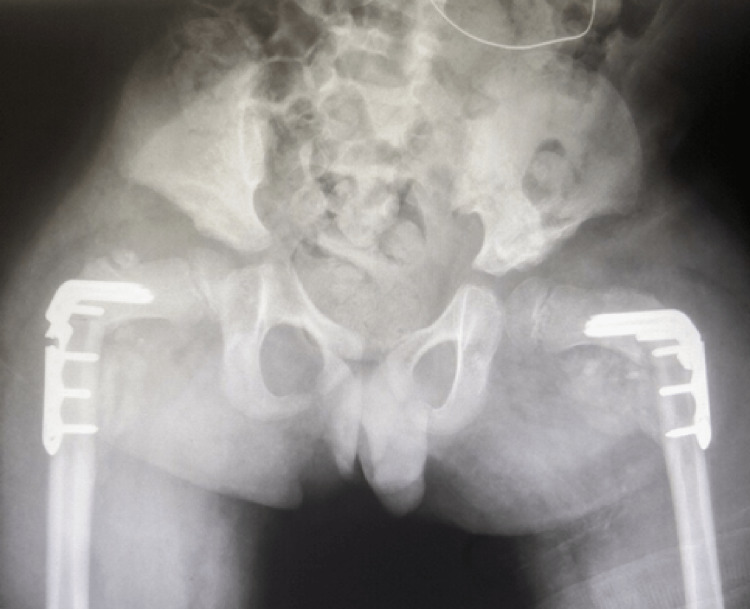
Four weeks postoperative AP radiograph of the pelvis demonstrating bilateral implant failure. AP, anterior-posterior
